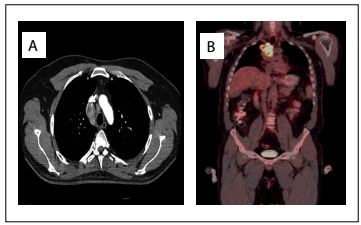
A) Computed tomography (CT) scan showing that the anterior mediastinal tumor invaded the major mediastinal structures. B) Positron-emission tomography (PET)-CT scan showing an aggressive mediastinal mass with high 18-FDG (fluorodeoxyglucose) affinity.
